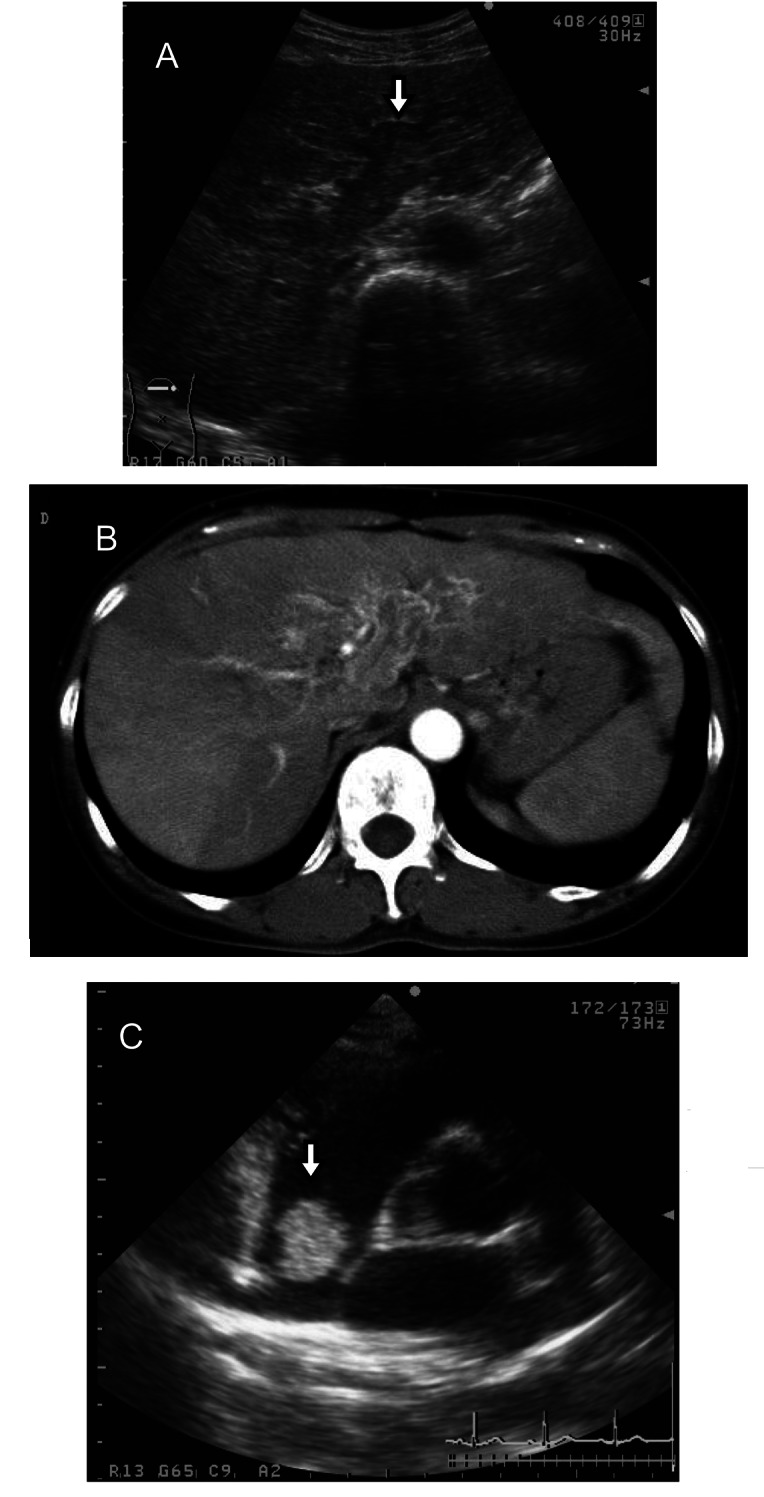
Imaging findings. (A) Abdominal sonographic image showing ill-defined large isoechoic mass with portal thrombus (arrow). (B) Computed tomography (CT) image showing ill-defined large heterogeneous mass occupying the bilateral liver lobes, and portal thrombus accompanying a cavernous transformation. (C) Transthoracic echocardiography image showing a round, movable tumor of a 2-cm diameter in the right atrium (RA; arrow).
